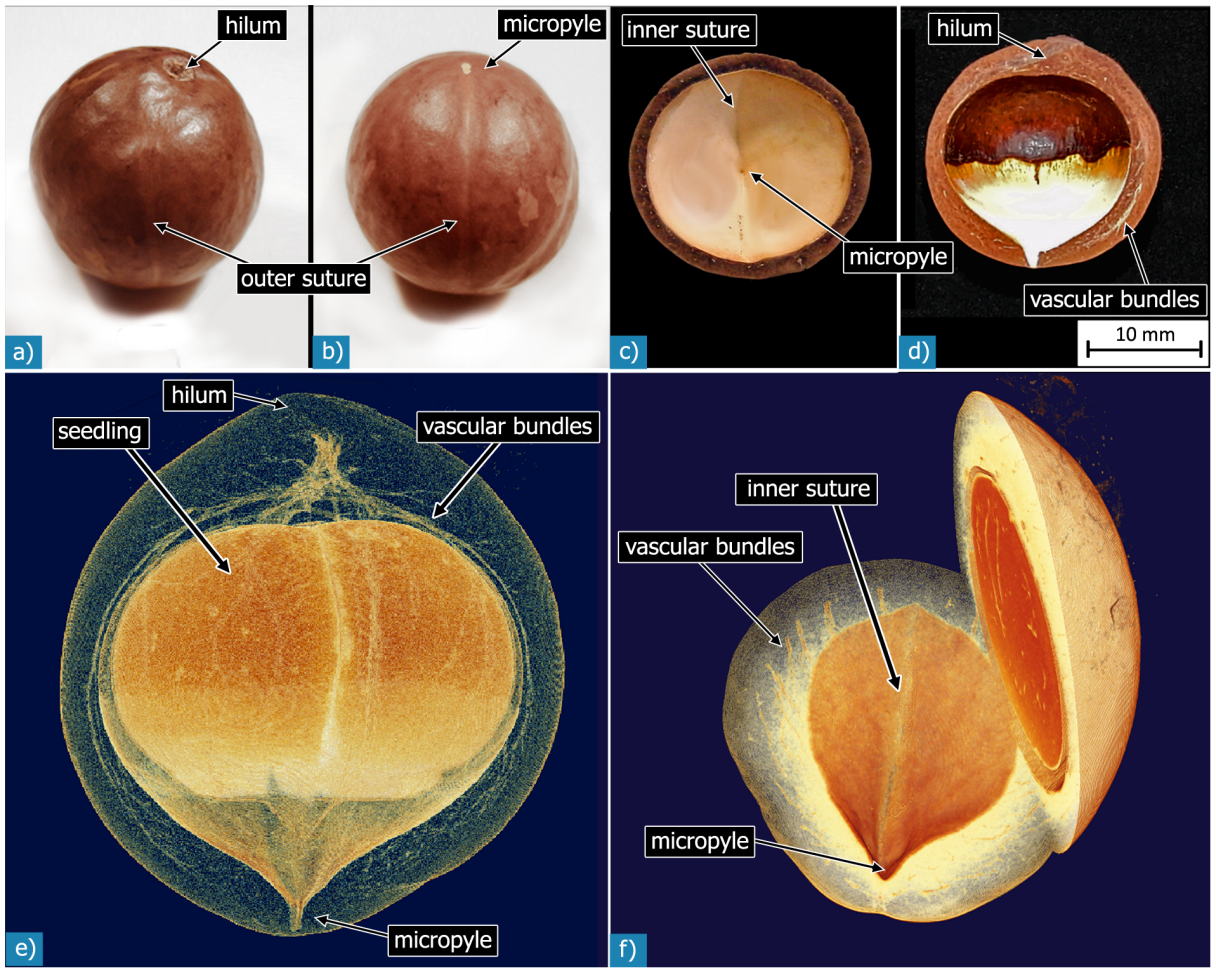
Photographs of an entire *Macadamia integrifolia* seed, showing the hilum, the micropyle and the outer suture (a, b). Light-brown speckles form an individual pattern on the surface of each shell (b). Photographs of seed coats cut normal (c) and parallel (d) to the outer suture show a locally varying thickness of the shell. The “normal” section (c) exhibits a nearly constant thickness, while the shell thickness of the “parallel” section (d) varies. The white structures within the coat material are the vascular bundles. Reconstructed 3D images from CT scans show the density, orientation and branching of the vascular bundles running from the hilum to the micropyle within the seed coat (e, f).
